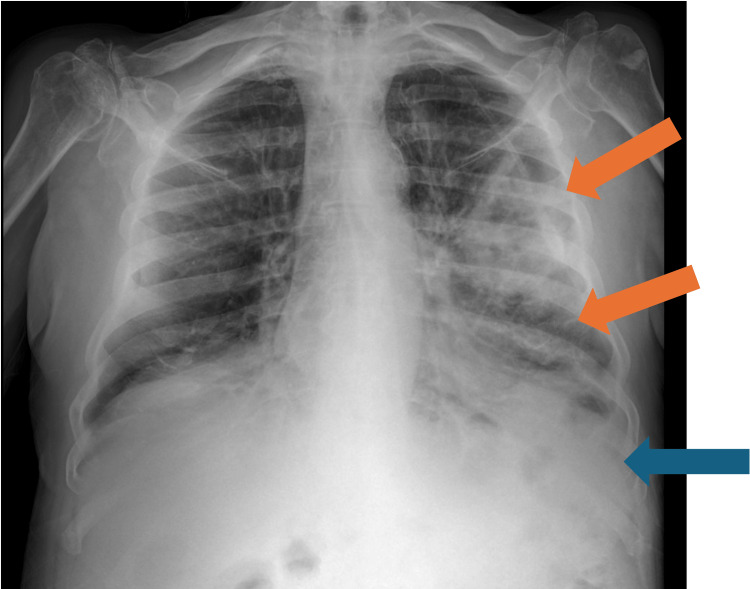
Chest X-ray at admission showing left-sided pneumonia (orange arrows) and pleural effusion (blue arrow)
